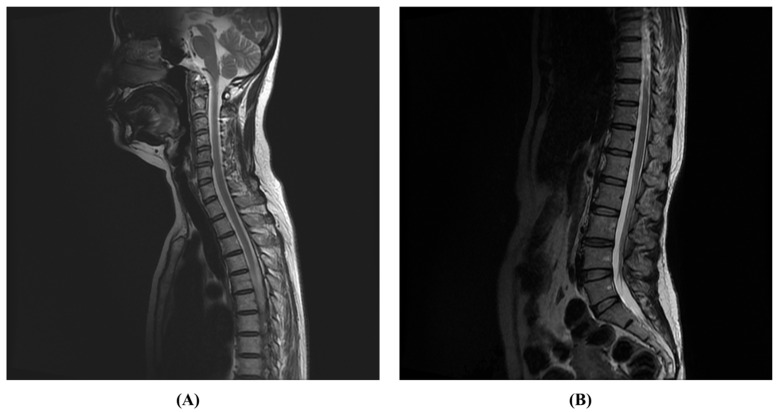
These figures are T2-weighted images of a spine MRI performed 7 months after onset. Compared to the previous MRI, it can be seen that longitudinally extensive transverse myelitis (LETM) findings from the T2 vertebral body segment to the T12 vertebral body segment disappeared. (A): Sagittal view on T2-weighted MRI imaging at the cervical and thoracic spine level, (B): Sagittal view on T2-weighted MRI imaging at the thoracic and lumbar spine level.
